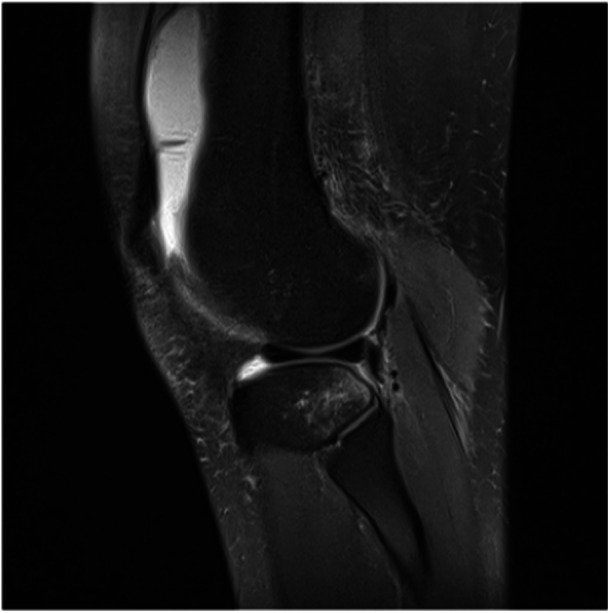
Magnetic resonance imaging scan with popliteofibular ligament tear.
